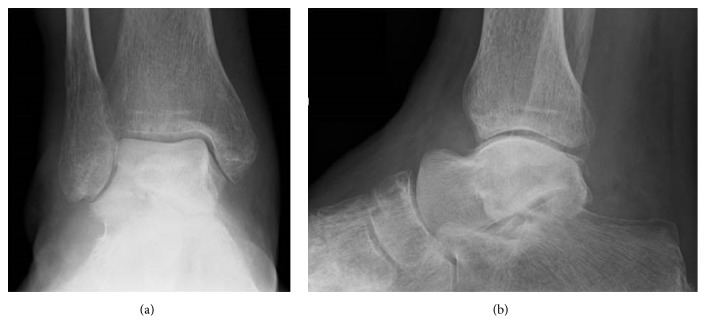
Preoperative radiographs showing sclerotic change and collapse of the talar body in anteroposterior (a) and lateral (b) views.
